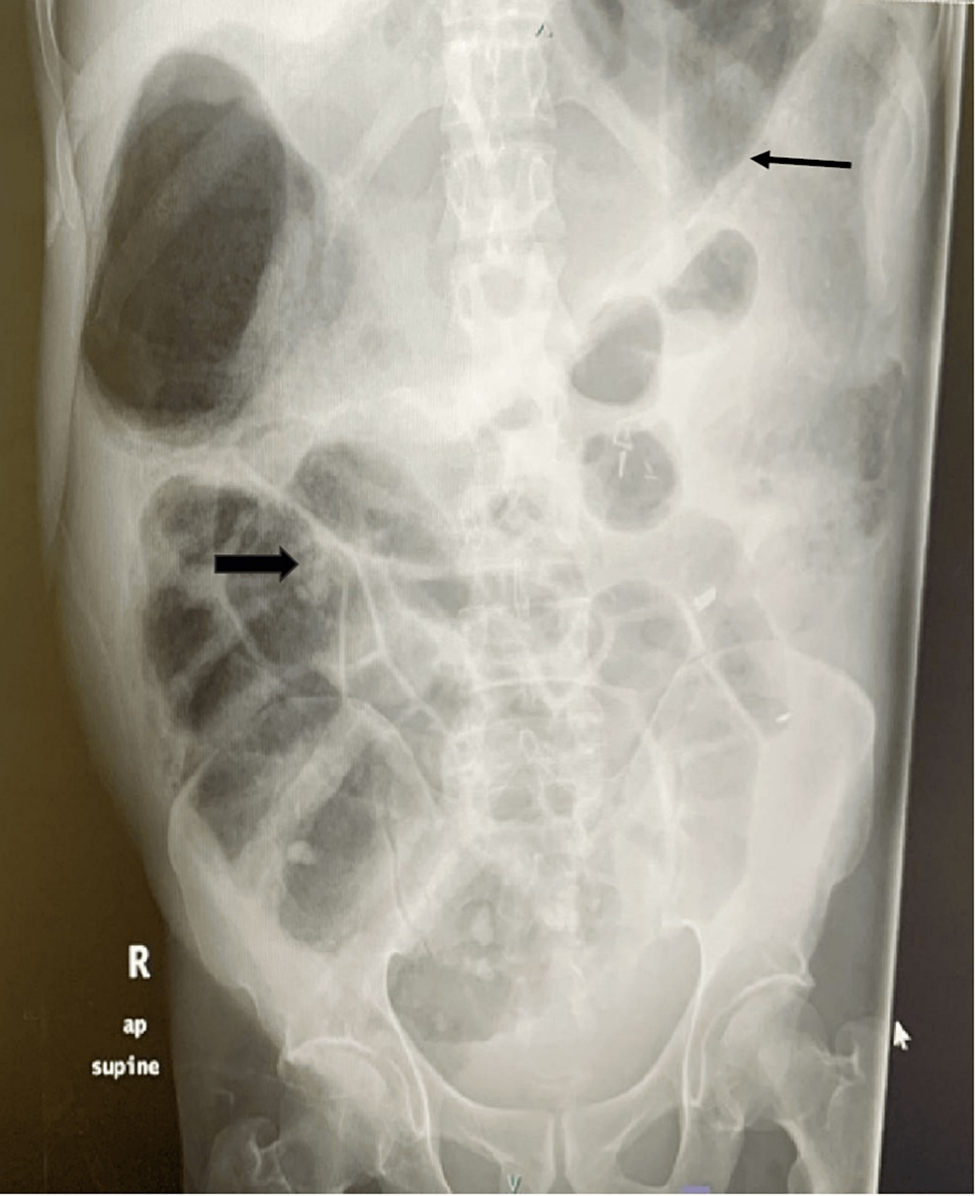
A plain abdomen (AP supine view) X-ray. The image shows the colon is loaded with fecal matter (block arrow) and grossly dilated, involving the ascending and transverse colon, reaching up to 9 cm in transverse (line arrow).
